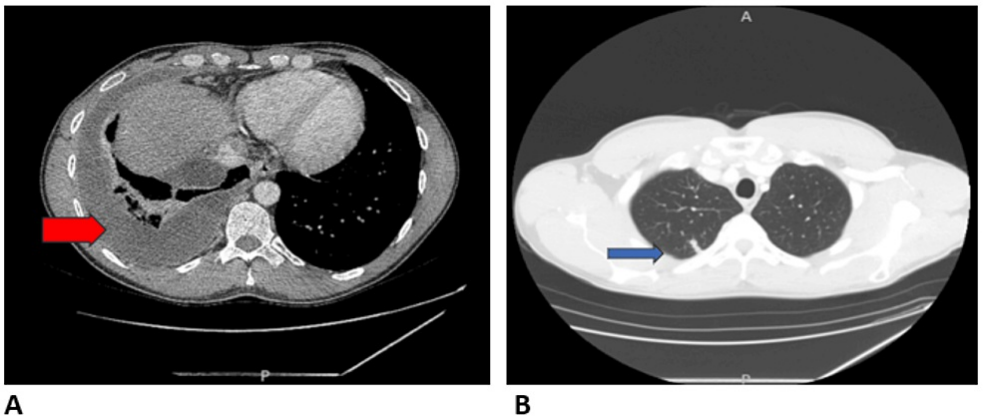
CT chest without contrast shows right pleural effusion and right upper lobe pulmonary nodule. The red arrow indicates right pleural effusion. The blue arrow indicates the right upper lobe pulmonary nodule. (A) The soft window shows the right pleural effusion (red arrow). (B) The lung window shows the right upper lobe pulmonary nodule (blue arrow).
